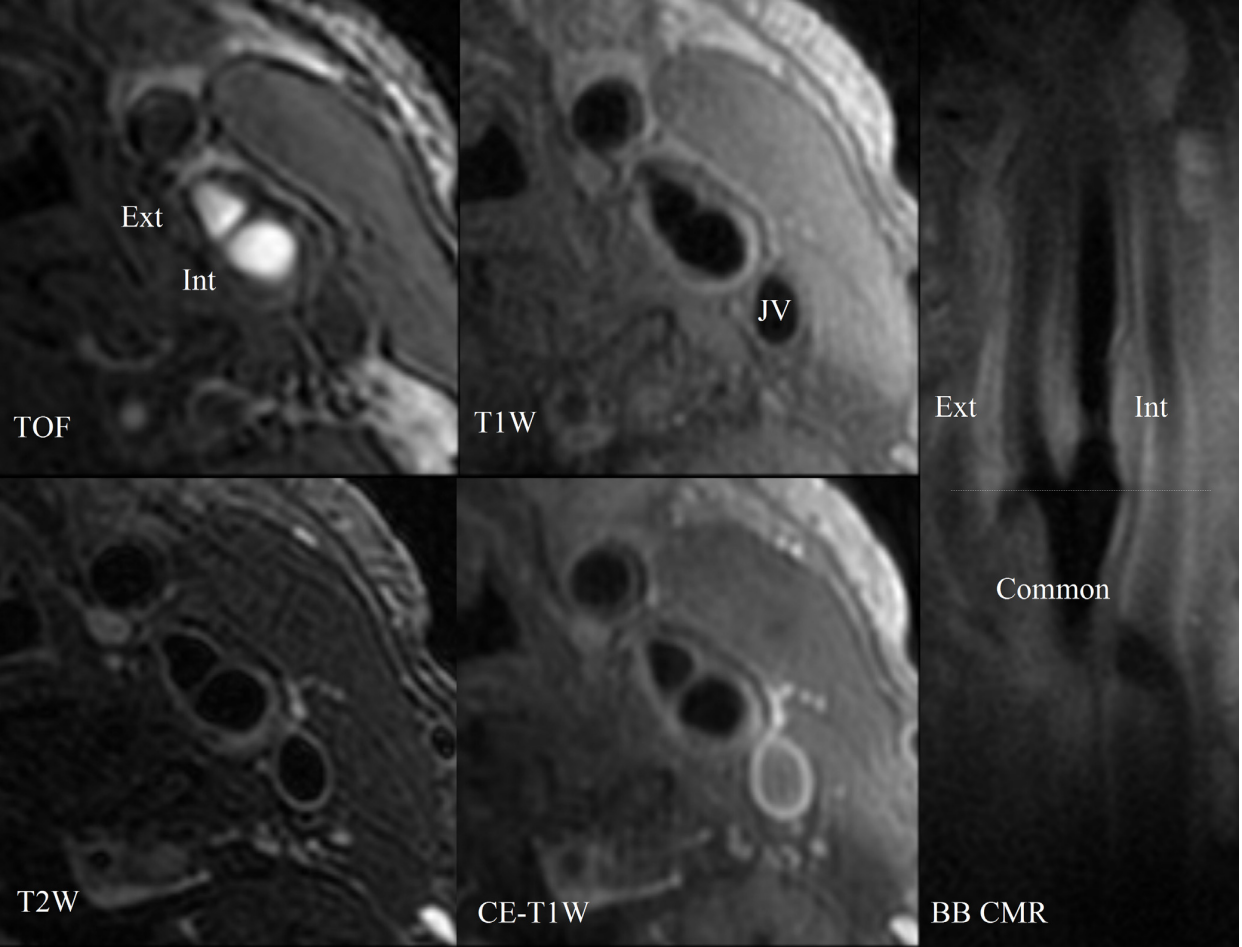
Example of carotid multisequence cross-sectional CMR images and a longitudinal black-blood CMR (BB CMR) angiography. Four contrast-weighted CMR images [3-dimensional time-of-flight (TOF), T1-weighted (T1W), T2-weighted (T2W), and contrast-enhanced T1-weighted (CE-T1W)] are matched using the carotid bifurcation (dashed line) seen on the longitudinal BB CMR angiography as the landmark. Proton density weighted image is not shown. Int = internal carotid artery. Ext = external carotid artery. Common = common carotid artery. JV = jugular vein.
